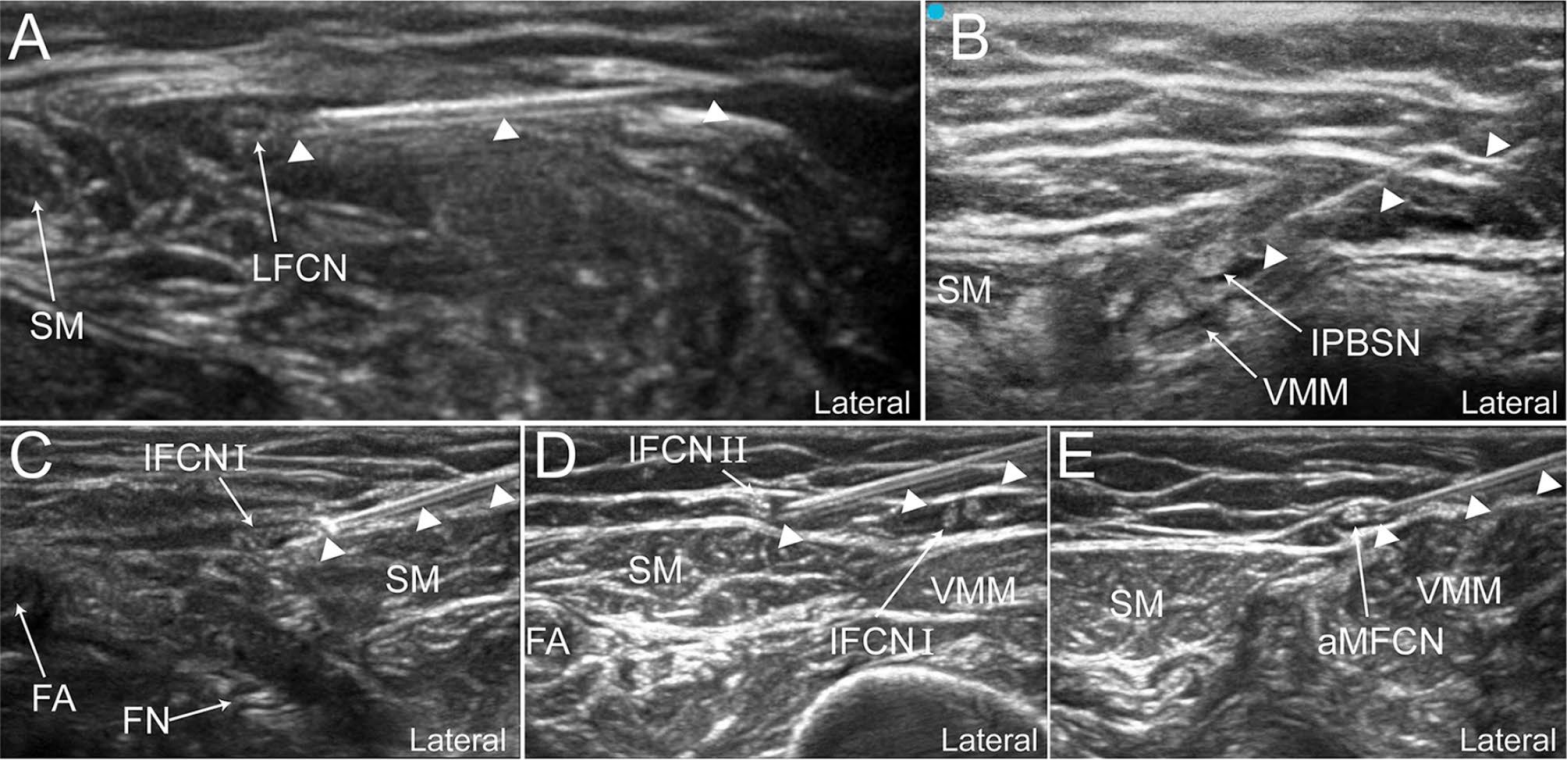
Ultrasound-guided dye injection of the PP in cadavers. A Dye injection of the LFCN; B Dye injection of the IPBSN; C Dye injection of the IFCN I; D, Dye injection of the IFCN II; E Dye injection of the aMFCN. SM, sartorius muscle; VMM, vastus medialis muscle; FA, femoral artery; FN, femoral nerve; LFCN, lateral femoral cutaneous nerve; IPBSN, infrapatellar branch of the saphenous nerve; IFCN I, intermediate femoral cutaneous nerve branch I; IFCN II, intermediate femoral cutaneous nerve branch II; aMFCN, anterior branch of the medial femoral cutaneous nerve. White triangles represent the needle trajectory
